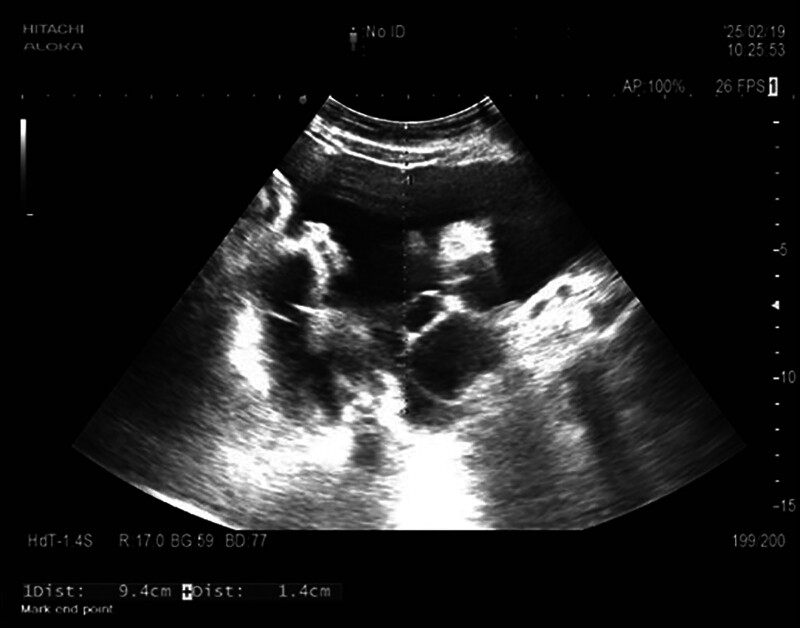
Abdominal ultrasound demonstrating ascites with a maximum depth of approximately 9.4 cm.
